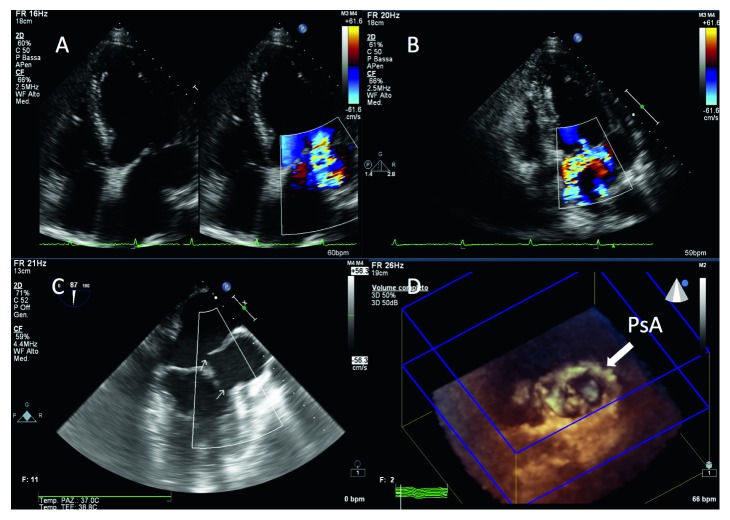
Transthoracic echocardiography showing the submitral pseudoaneurysm (A); at color Doppler, communication between the false chamber and left atrium is evident (B); transesophageal echocardiography displayed the false chamber communicating with both the left atrium and left ventricle (C, arrows); 3D transthoracic echocardiography displaying the left atrium and the false chamber (PsA) paired (D).
